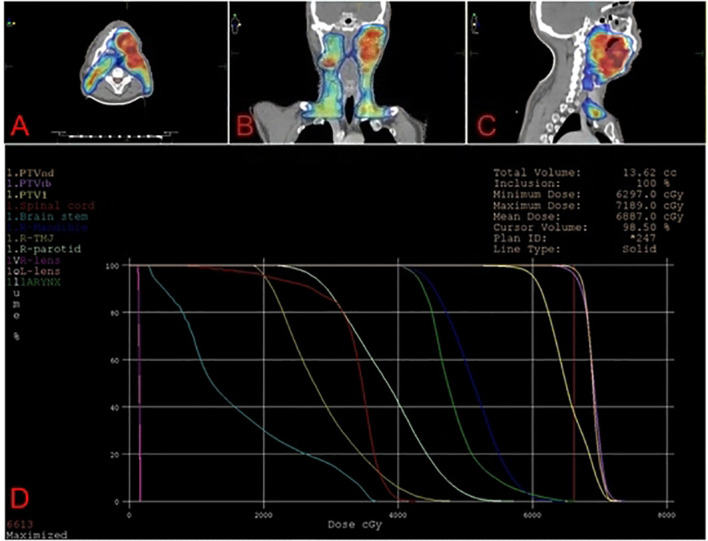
Radiotherapy dose distribution and planning evaluation. (A-C) The 95% isodose line encompassing the planned target volumes is displayed on pretreatment planning CT images in the (A) axial, (B) coronal, and (C) sagittal planes. The target volumes are color-coded as follows: tumor bed clinical target volume (PTVtb, purple), nodal clinical target volume (PTVnd, brown), and high-risk clinical target volume (PTV1, yellow). (D) The dose-volume histogram.
